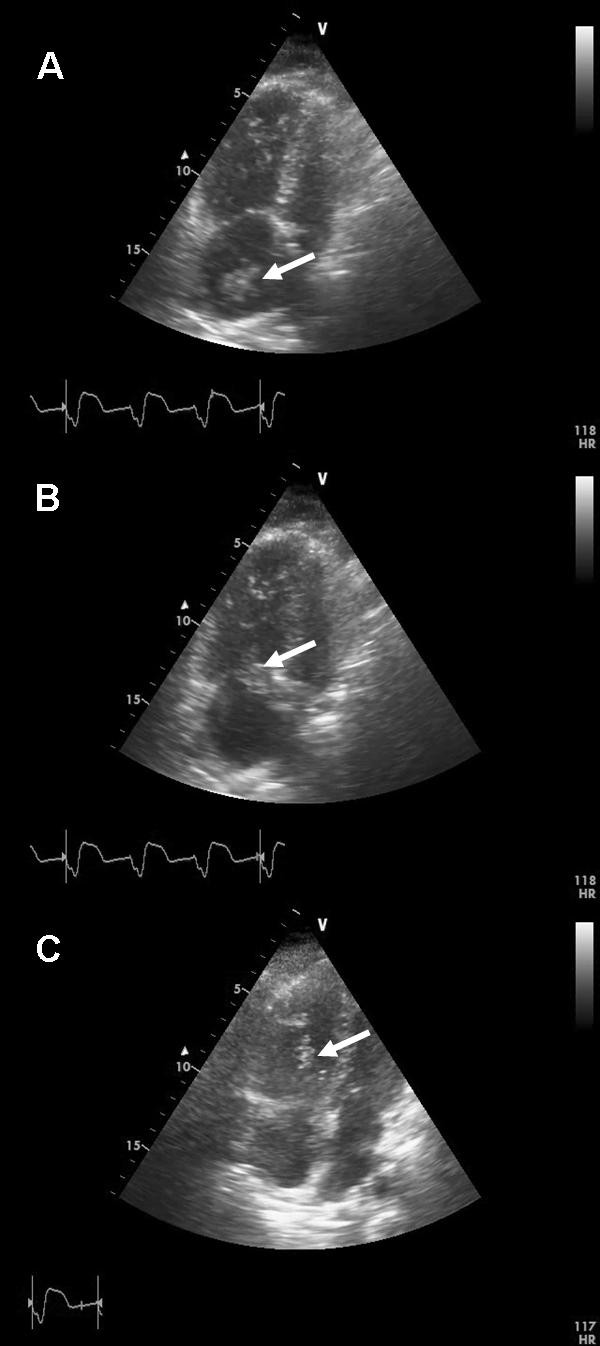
Transthoracic echocardiogram (TTE) with a right sided heart thrombus. Apical 4-chamber view with (A) a mobile thrombus (marked by arrow) in the right atrium moving through (B) the tricuspid orifice into (C) the right ventricle.
